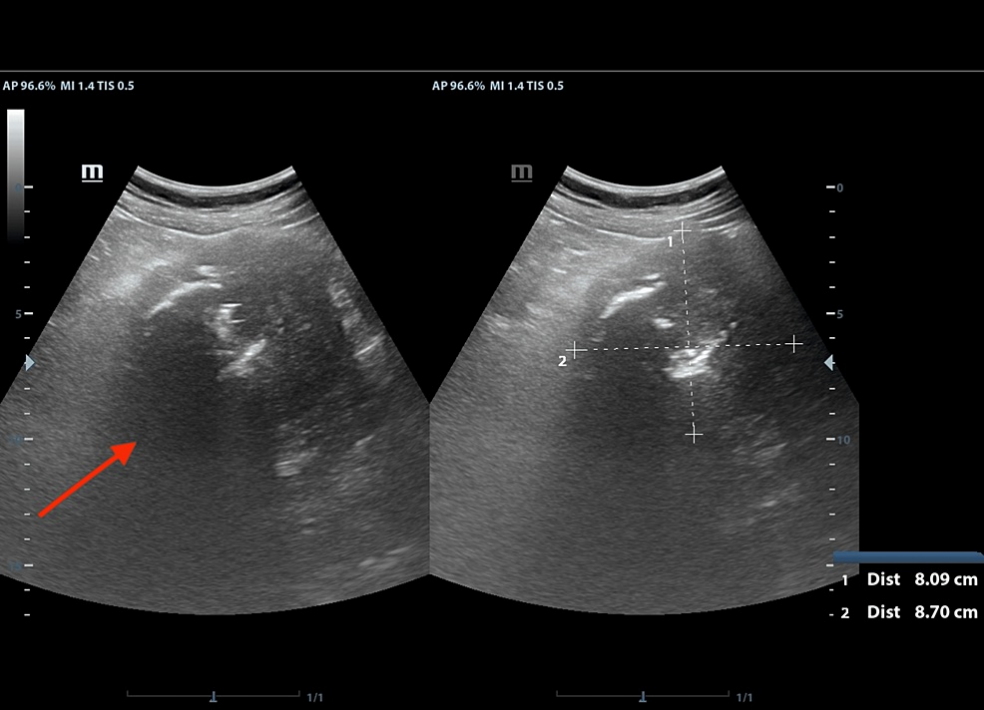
Ultrasound appearance of the lesion Note the gross calcifications and the heterogeneity of the lesion. The deep borders of the mass are indiscernible
